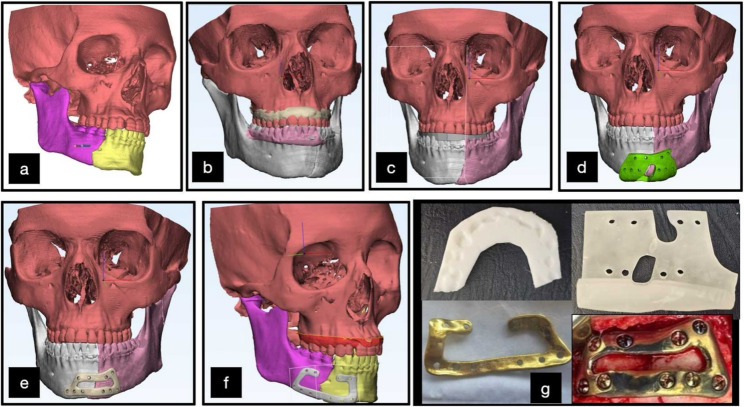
Virtual planning and custom component design. a Segmentation of the mandible from preoperative CT (threshold 226–3071 HU). b Virtual fracture reduction with occlusal verification; green indicates acceptable contact (< 0.1 mm, pragmatically defined). c 3D printed occlusal stent spanning both dental arches. d Surgical guide with cylindrical drill sleeves (inner diameter 1.8 mm). e Open superior PSI design (gap in superior component to bypass mental nerve) — used in Cases 1–2. f Closed PSI design (continuous box construct) — used in Cases 3–6. g The printed occlusal stent, surgical guide, and the milled PSIs
